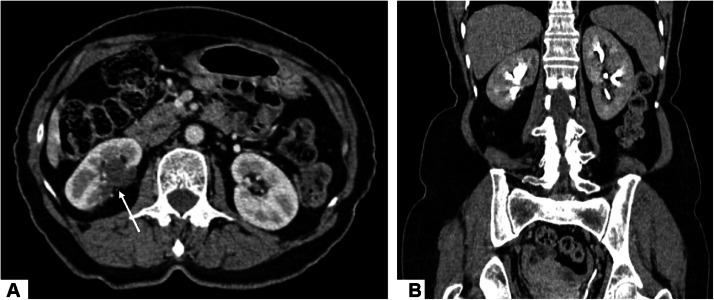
One-year follow-up contrast-enhanced CT: axial image in the portal phase (A) and coronal image in the delayed phase (B), showing a normal postoperative appearance of the right renal tumorectomy site (arrow). The renal parenchyma enhances homogeneously, with no residual lesion, and the kidney demonstrates normal contrast excretion.
